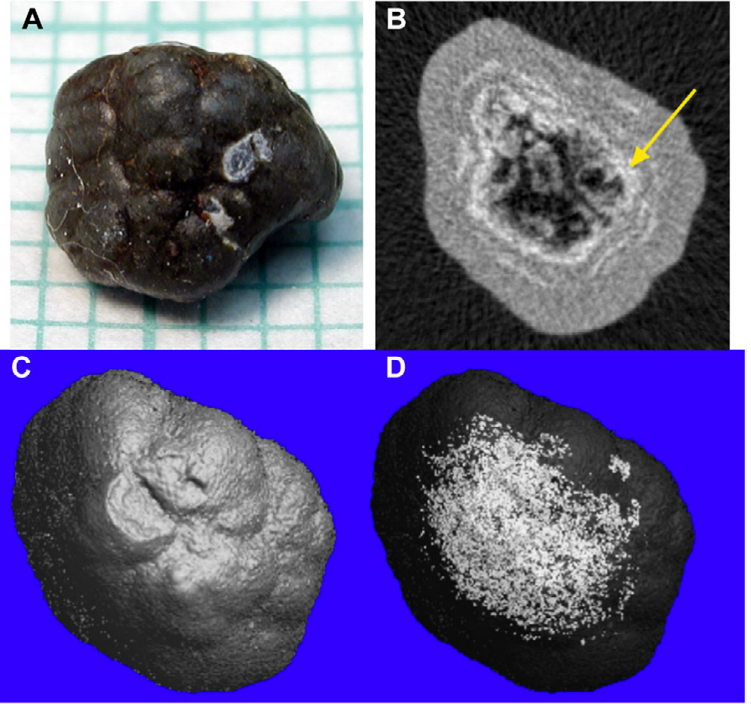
Visualization of components of a mixed COM/HA stone. A. Digital photograph of stone, on 1 mm grid. B. Micro CT slice through middle portion of stone; arrow indicates ring of high attenuation (hydroxyapatite). Gray material outside this ring had attenuation value in the range for COM. C. 3-D surface rendering from micro CT displaying surface topology. D. 3-D surface rendering with transparent shell to show 3-D localization of hydroxyapatite within stone.
